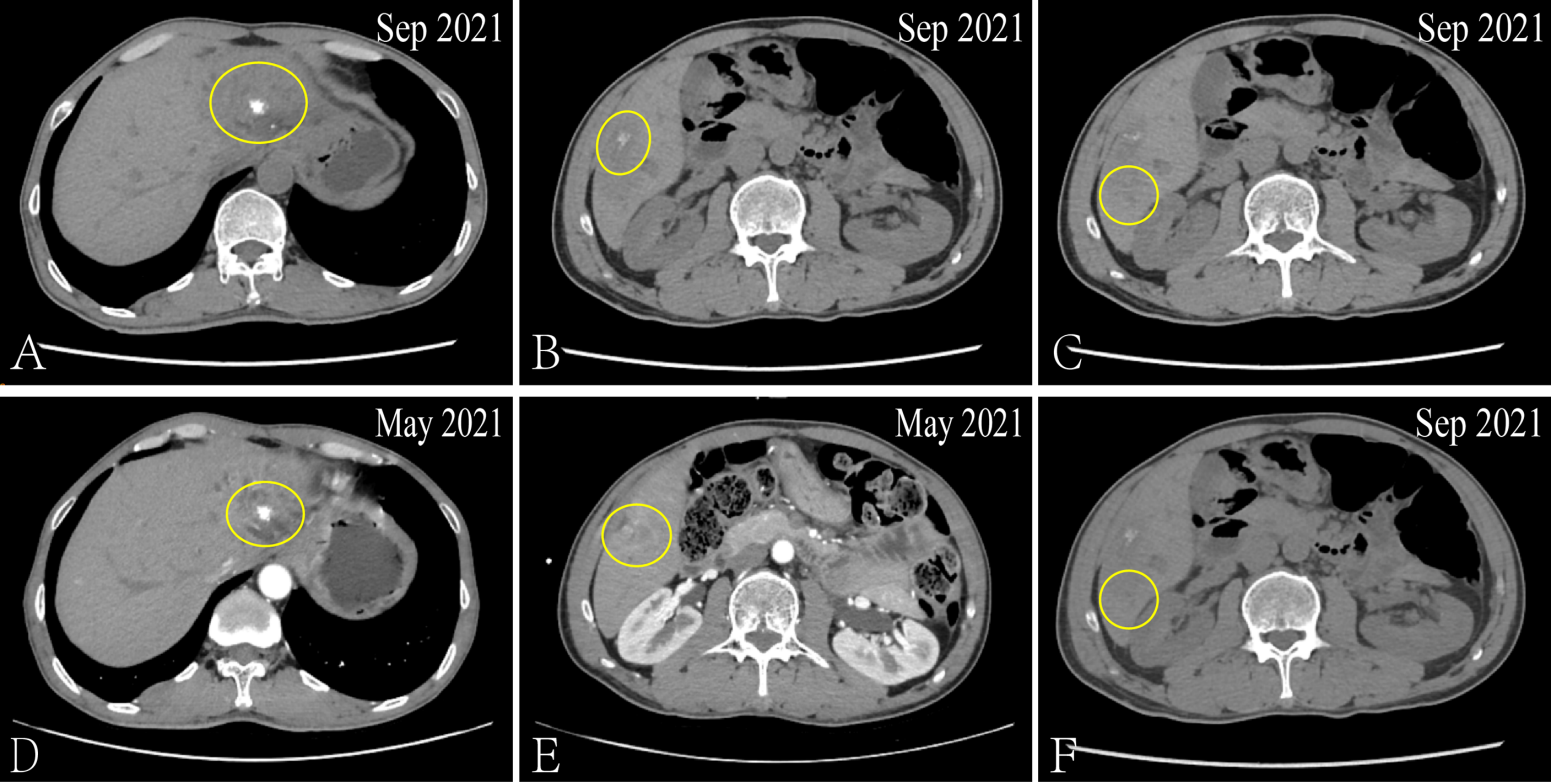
Via* CT to contrast the curative effect between the Sep 2021 and May 2021. The size of the metastatic lesion which in the left and caudate lobe of liver is increase from 2.6 × 2.1cm to 3.3 × 2.6cm (A, D). The size of the metastatic lesion which in liver S5 is reduction from 2.7 × 1.7cm to 2.3 × 1.1cm (B, E). The new metastatic lesion (1.5 × 1.2cm) in liver S6 (C, F).
